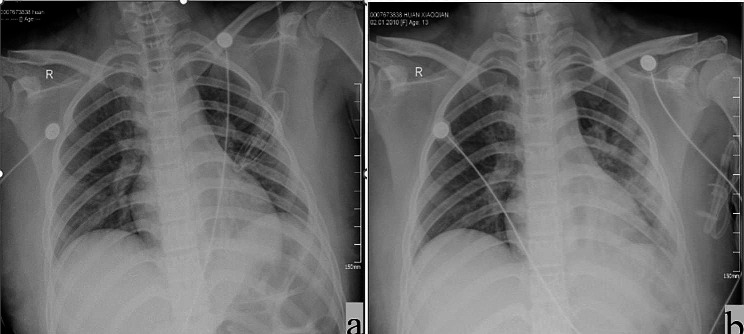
Chest radiograph results: a Case B on day 5 of admission, revealing no obvious abnormalities. b Case B on day 17 of admission, revealing obvious exudation from the left lung
